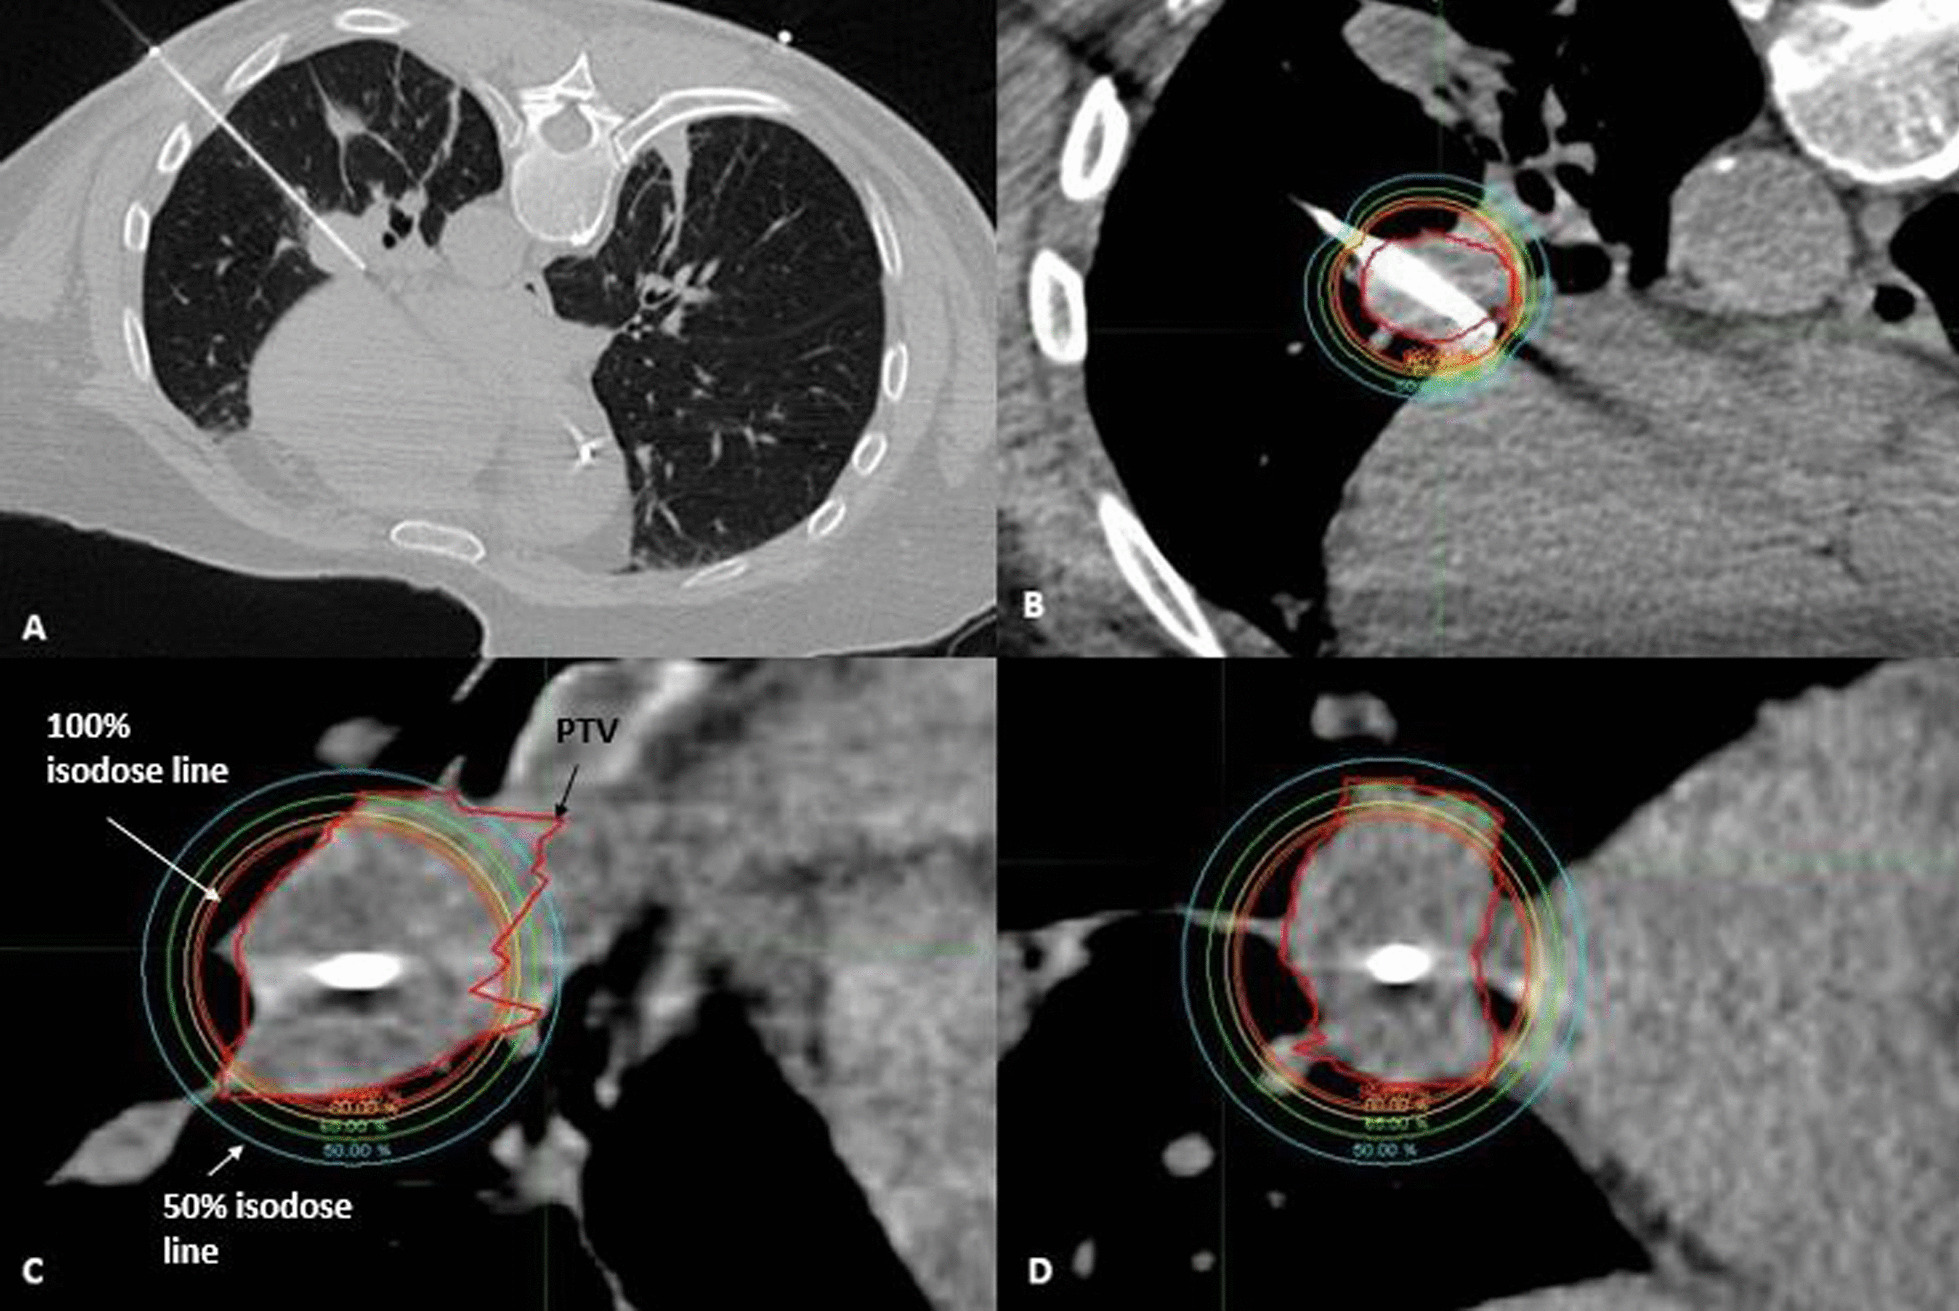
A 60-year old man with metastatic leiomyosarcoma presenting with multiple lung metastasis. He had undergone multiple microwave ablations for other lung tumors. A malignant left sub-hilar lymph node continued to grow despite treatment with multiple cycles of doxorubicin and olaratumab. This tumor was treated with CT-guided interstitial HDR brachyablation. A Placement of co-axial needle under CT-guidance during brachytherapy catheter implantation. A co-axial needle was advanced percutaneously and placed directly into the ultra-central tumor abutting the heart. B Axial C Sagittal and D Coronal views of resultant treatment isodose distribution
